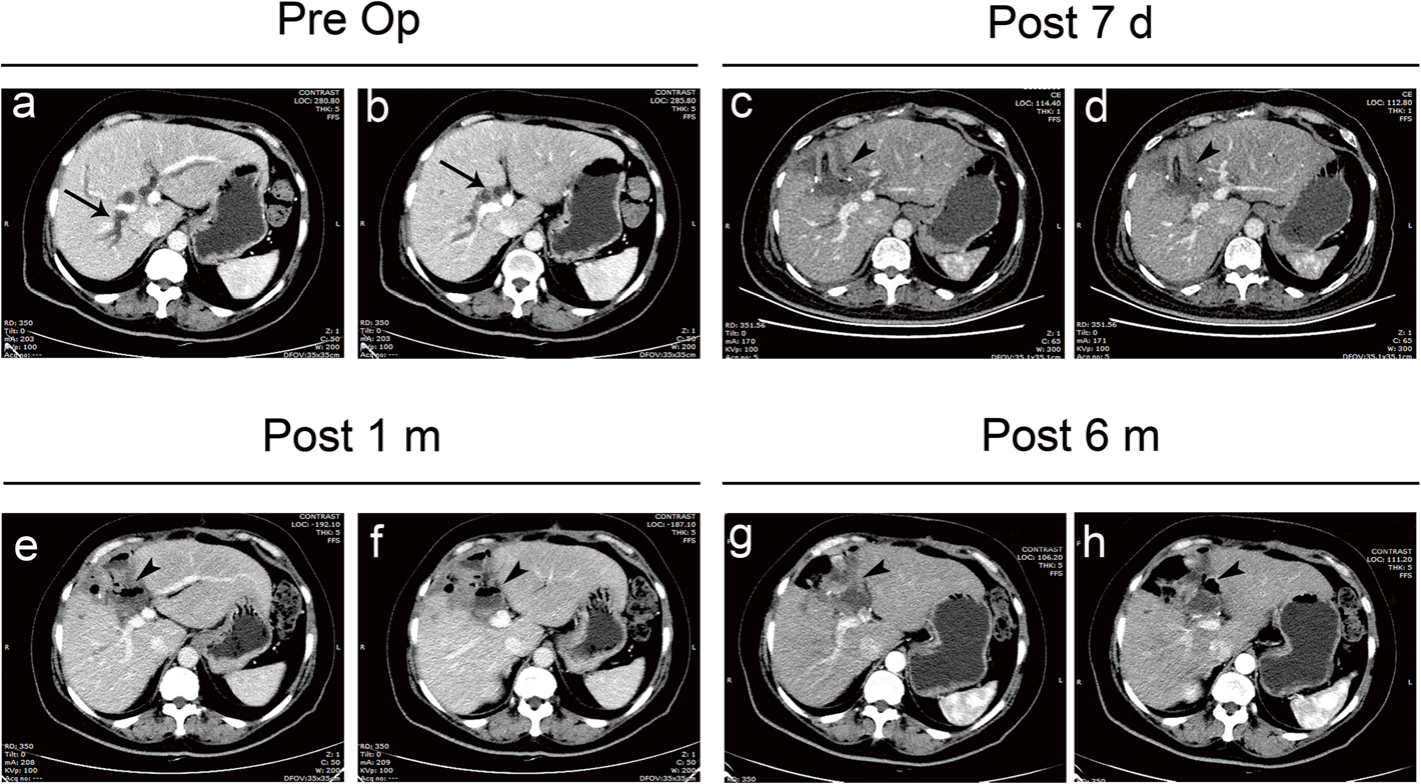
The CT scans of the No.1 patient before and after the operation. (a, b) The dilated bile ducts before surgery (arrow); (c, d, e, f, g, h) The dilated bile ducts had restored to normal after the operation, and the anastomotic stoma healed well without stenosis (arrowhead). Pre OP: pre-operation; Post 7 d: post-operation 7th day; Post 1mon: post-operation 1st month; Post 6mon: post-operation 6th month
